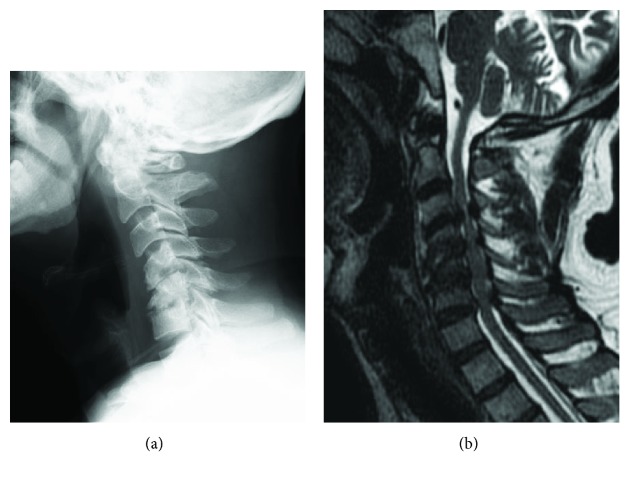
(a) X-ray of the cervical spine obtained just before the initial surgery showed destructive changes of the cervical spine, with a C2-7 lordotic angle of 0°. (b) Sagittal T2-weighted MRI of the cervical spine performed just before the initial surgery showed multisegmental stenosis at the C3/4, C4/5, C5/6, and C6/7 disc levels.
